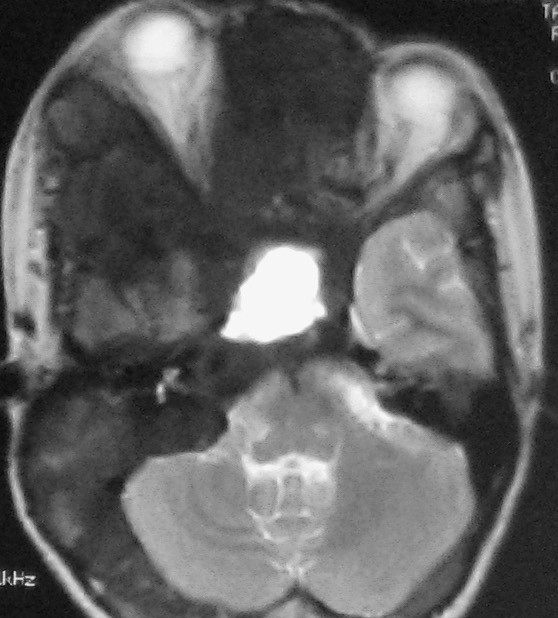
Axial T2-weighted MR images show abnormal thickening of the bone involving the frontal bone, sphenoid bone, lateral and medial orbital walls, with right canal is shallow and optic nerve is compressed.
